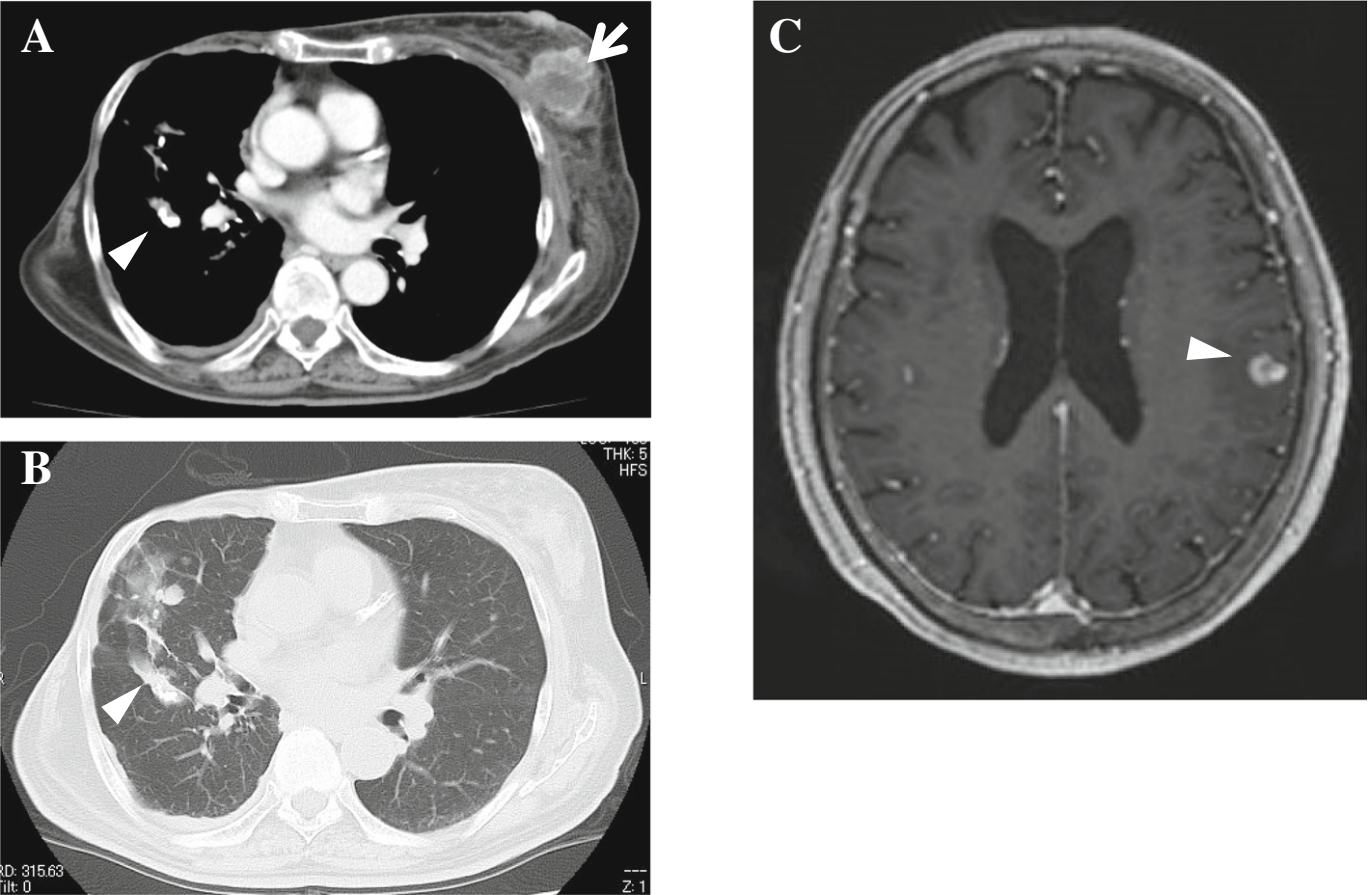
a Contrast-enhanced computed tomography (CT) mediastinal window showing the suspected breast cancer on the left side (*arrow*) and lung metastasis (*arrowhead*). b Plain CT lung window showing the suspected lung metastasis (*arrowhead*). c Axial magnetic resonance imaging scan of the brain with T1-weighted gadolinium contrast enhancement showing one small coin lesion in the temporal lobe of the left side with a diameter of about 5 mm, a suspected metastasis (*arrowhead*)
